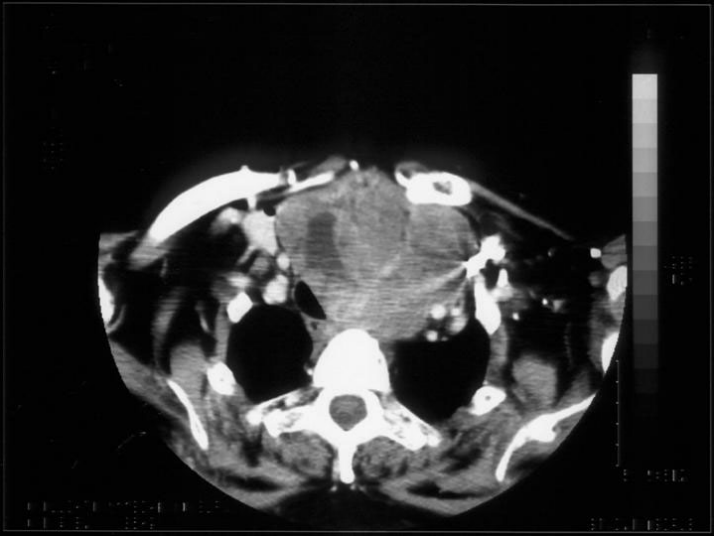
CT Scan showing a large solid left neck mass, with extension to the mediastinum and displacement of the trachea, oesophagus and great vessels.
